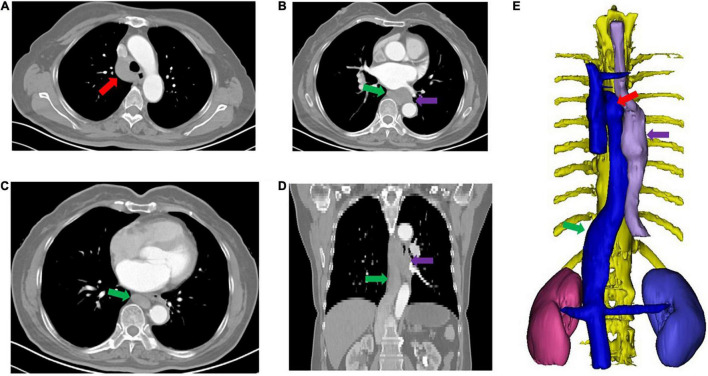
CT reconstruction showing azygos continuation of the inferior vena cava (not preoperatively recognized). (A–D) Show the enlarged arch of the azygos vein and azygos vein. (E) Shows hepatic segment absence of the inferior vena cava with azygos continuation. The red arrow shows the enlarged arch of the azygos vein; the green arrow shows the azygos vein; the purple arrow shows the tumor.
